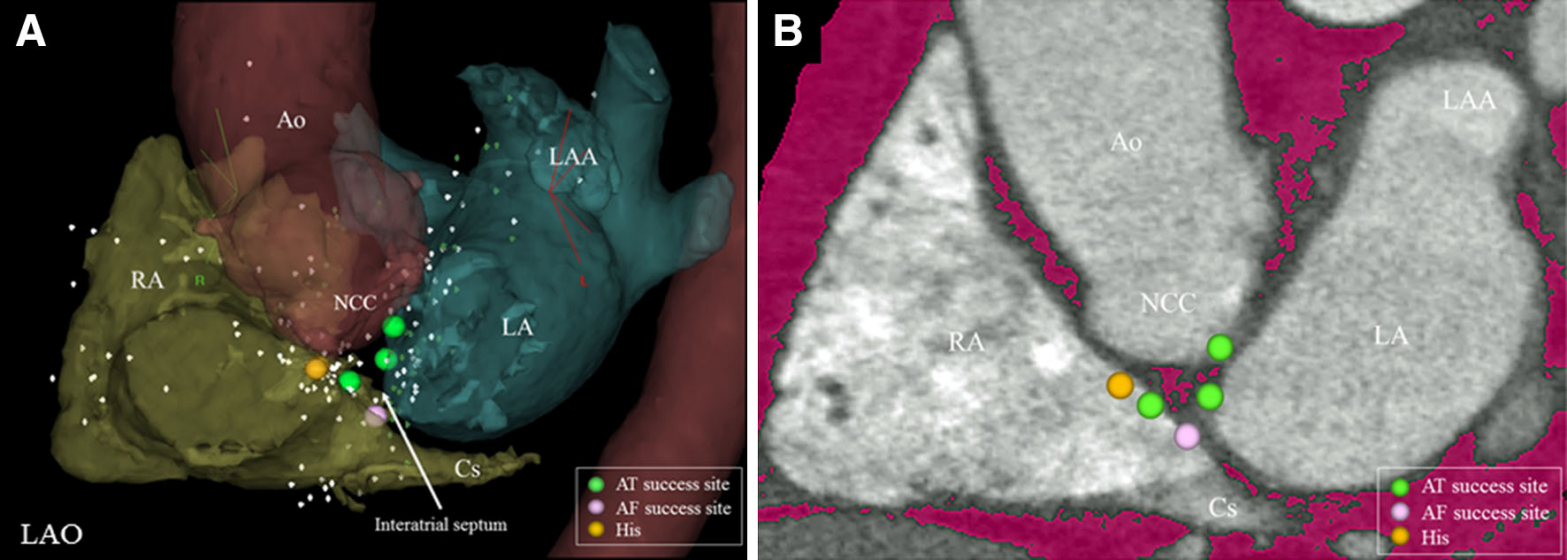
Successful ablation sites (AT: *green tag*, AF: *pink tag*) in the atrial septum near the atrioventricular node on an electroanatomic map (a) and the corresponding short-axis computed tomography image (ZioM900; Ziosoft, Tokyo, Japan) (b). *Color maps* were overlaid on CT images to mark fat-density pixels with *red color* (fat defined based on Hounsfield units between −180 and 0). Intramural fat deposition is clearly demonstrated in the interatrial septum (*red color*). *RA* right atrium, *LA* left atrium, *LAA* left atrial appendage, *Ao* aorta, *NCC* noncoronary aortic cusp, *Cs* coronary sinus, *LAO* left anterior oblique
